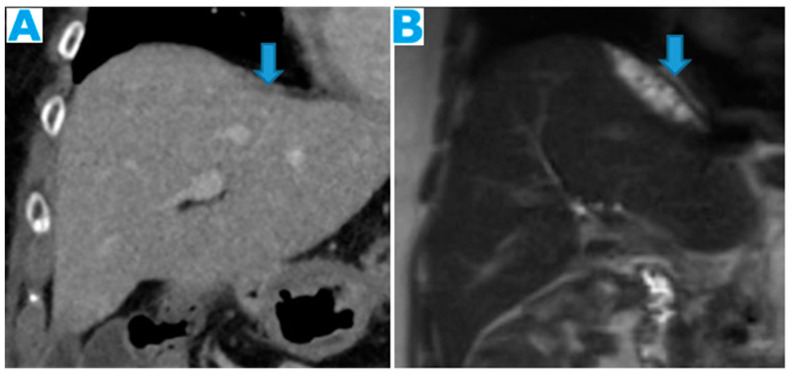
Coronal T2WI ((A), from five years prior) and CE-CT coronal MPR ((B), current follow-up). PC from ovarian granulosa cell tumour: Notice, in (B), a deposit within the right subphrenic space, bulging into the hepatic capsule (arrow). See how subtle it originally was in (A) (arrow); it could easily be overlooked upon axial imaging.
